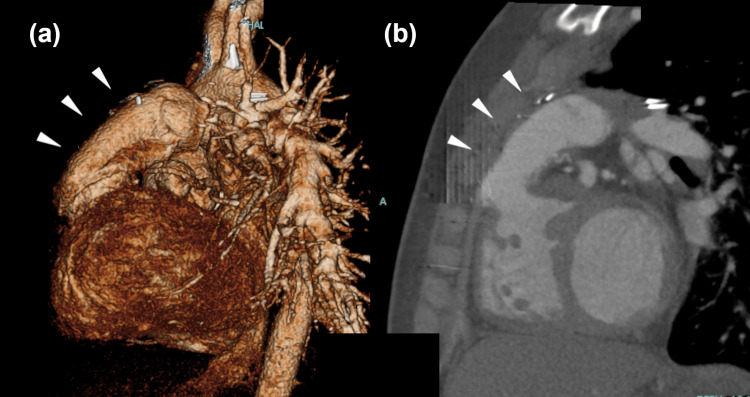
Computed tomography images three months after re-reoperation: (a) Volume rendered image, left side view; (b) similar sagittal image. Note the smooth and severe flexion-free form of conduit (arrows).
